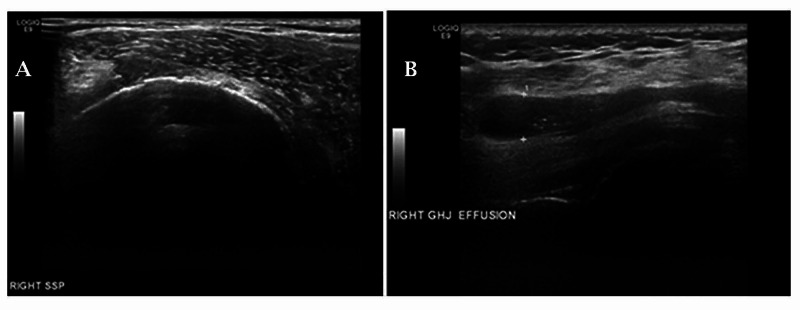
Ultrasound scan of the right shoulder A. Bare humeral head suggestive of rotator cuff tear; B. Effusion of the glenohumeral joint
